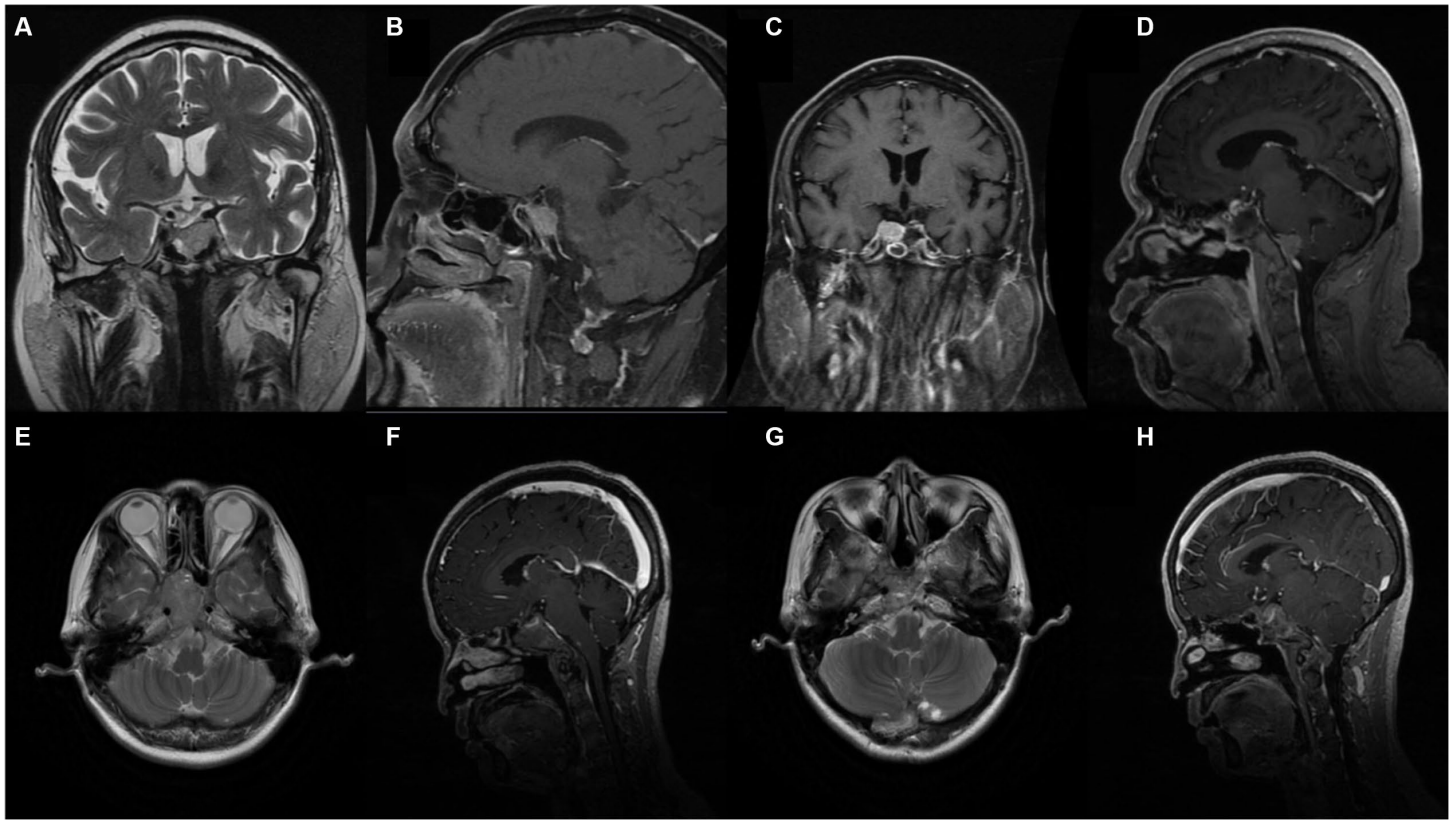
Pre-operative enhanced Magnetic Resonance Imaging (MRI) revealed large mass lesion in the sellar region. (A, B) Case 1. Pre-operative MRI demonstrated large tumour in the pituitary gland involving left sinus. (C, D) Case 1. Post-operative enhanced MRI showing decreases tumour mass and cancer metastasis. (E, F) Case 2. Pre-operative MRI with heterogeneous enhancement. (G, H) Case 2. Post-operative MRI.
